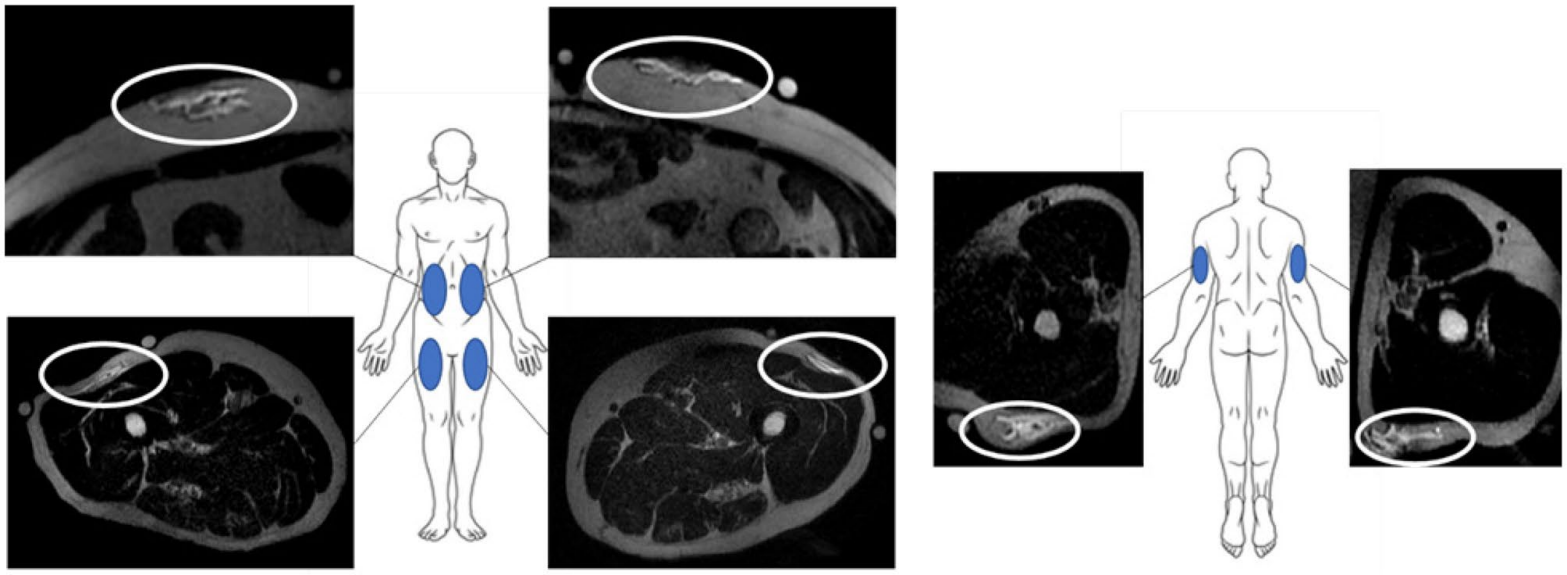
LVSC injection site locations. LVSC injection sites include abdomen (upper left), thigh (lower left), and arm (right) with representative MRI axial images showing saline depots (white ovals) at peak injection volume. For abdomen and thigh, incremental delivery was administered up to 10 mL and up to 5 mL in the arm sites. Reference anatomy including bony structures, organs, and soft tissue planes are clearly visible. Permission was obtained to use and modify the human graphic (copyright: Sergey Tkachevto, Dreamstime.com)
